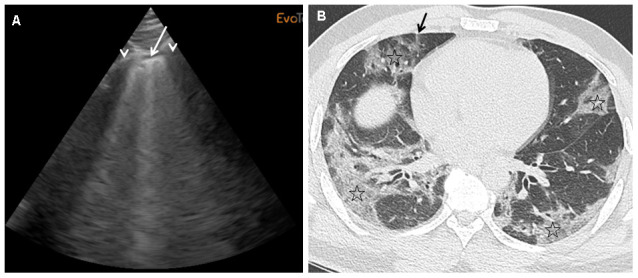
(A)This panel shows a grade 2 LUS image for a 45-year-old man at 10 days from initial symptoms, requiring oxygen (6 l/min), with a severity index of 0.58. On this sagittal ultrasound view encompassing a right anterior inter-rib space (arrows: rib shadows), one can see a typical lung rocket (arrow) as breath moving comet-tail artefact. (B) This panel shows an axial image of the corresponding CT scan performed at admission with typical COVID-19 interstitial pneumonia combining septal thickening (arrow) and peripheral ground glass opacities (stars).
